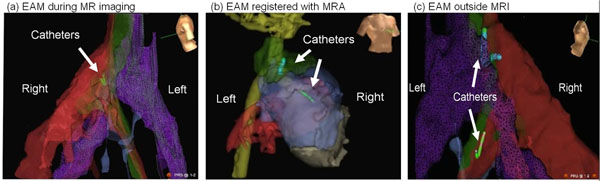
EAM allows catheter navigation inside, outside MRI and on segmented heart MRA
